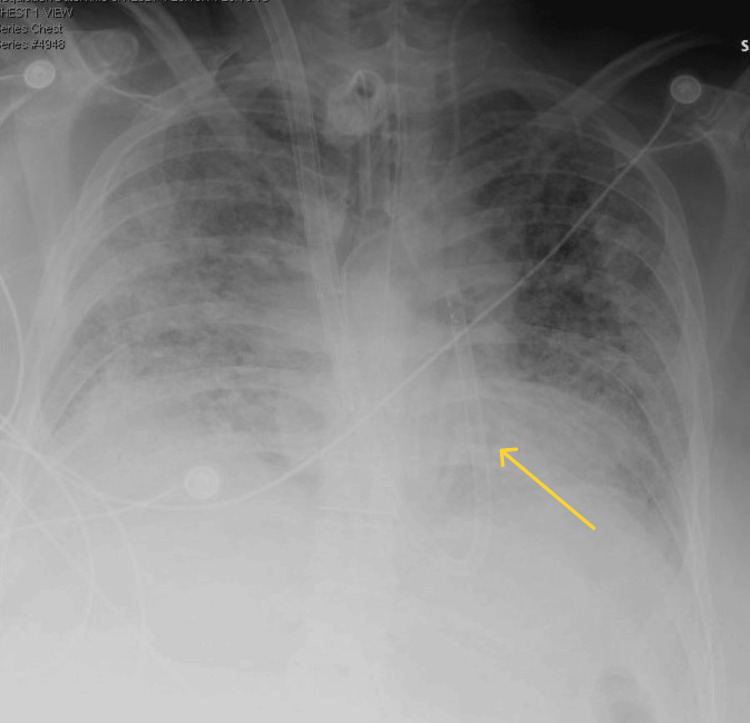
Chest X-Ray The yellow arrow indicates diffuse bilateral parenchymal airspace opacity concerning Acute Respiratory Distress Syndrome (ARDS) versus pulmonary edema. Stable cardiac and mediastinal contours. Small bibasal pleural effusions. No pneumothorax.
